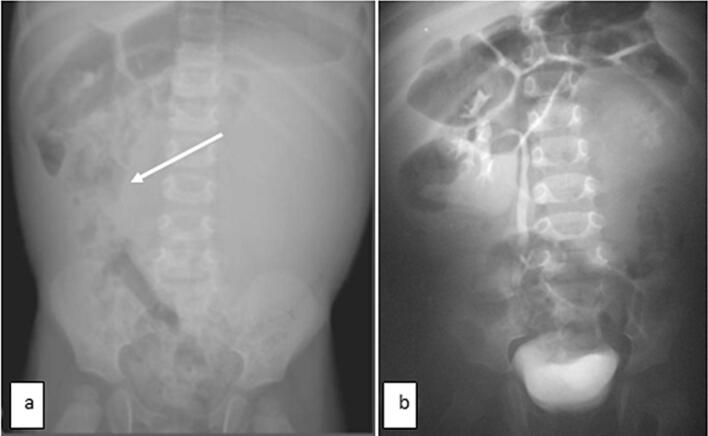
Radiological explorations. (a) Abdominal radiograph showing a left abdominal opacity that displacements the adjoining bowels (white arrow) (b) Intravenous pyelogram showing delayed left renal function with normal functioning right kidney.
